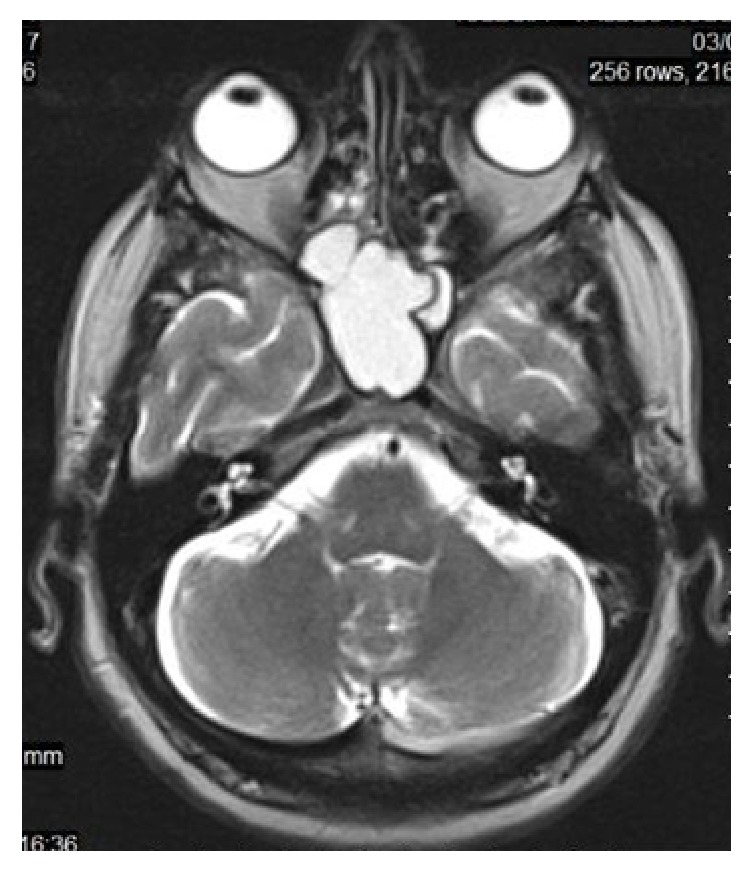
The lesion appearing hyperintense on T2-weighted RMI.
